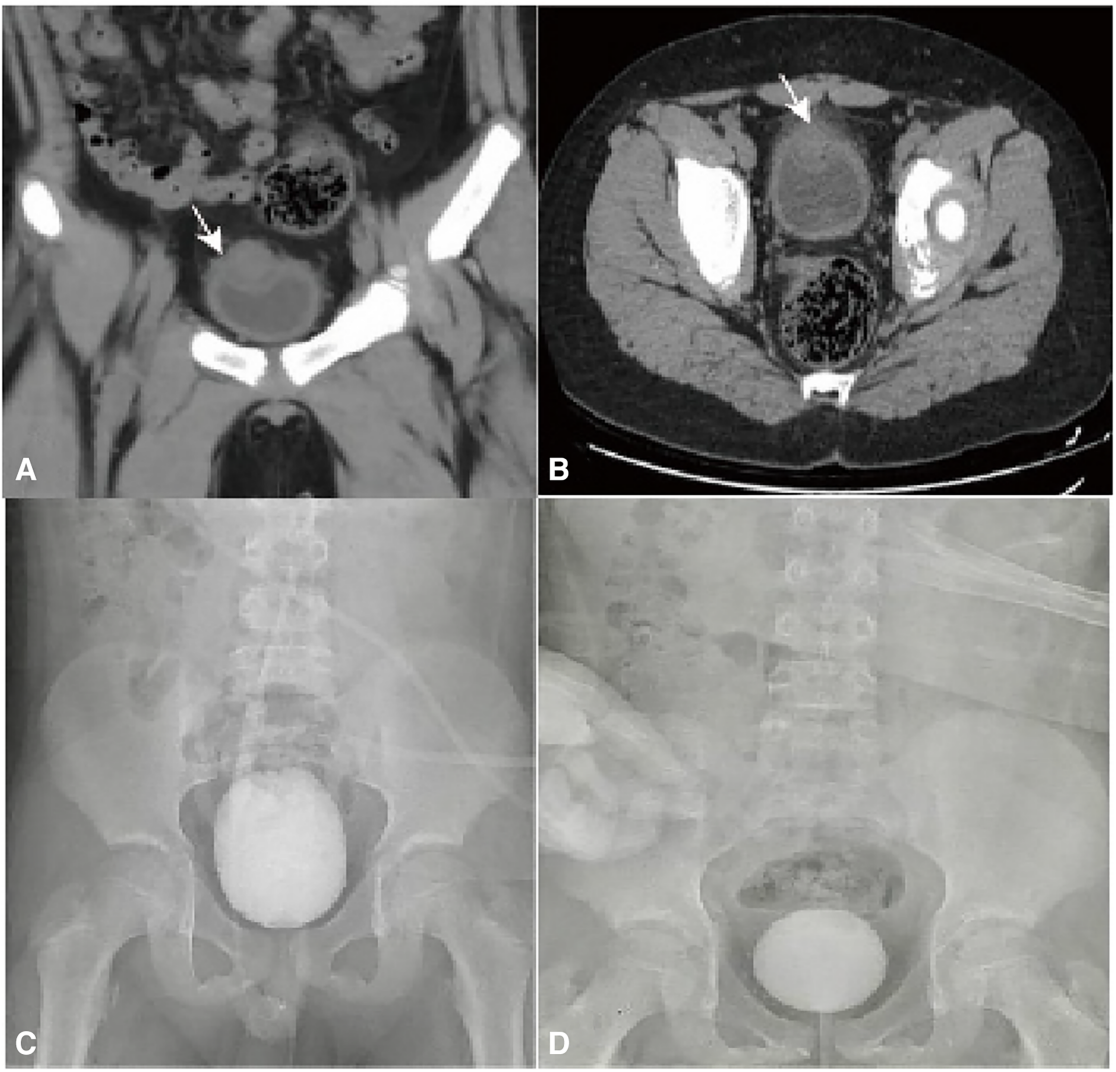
(A,B) CT scan revealed a mass of soft tissue density in the urachal area at the anterior upper edge of the bladder, approximately 32 mm × 33 mm × 29 mm in size, with unclear boundary with the bladder (arrow). (C,D) VCUG findings: No filling defects or diverticula were observed during bladder filling and emptying, and there was no reflux in the bilateral ureters.
